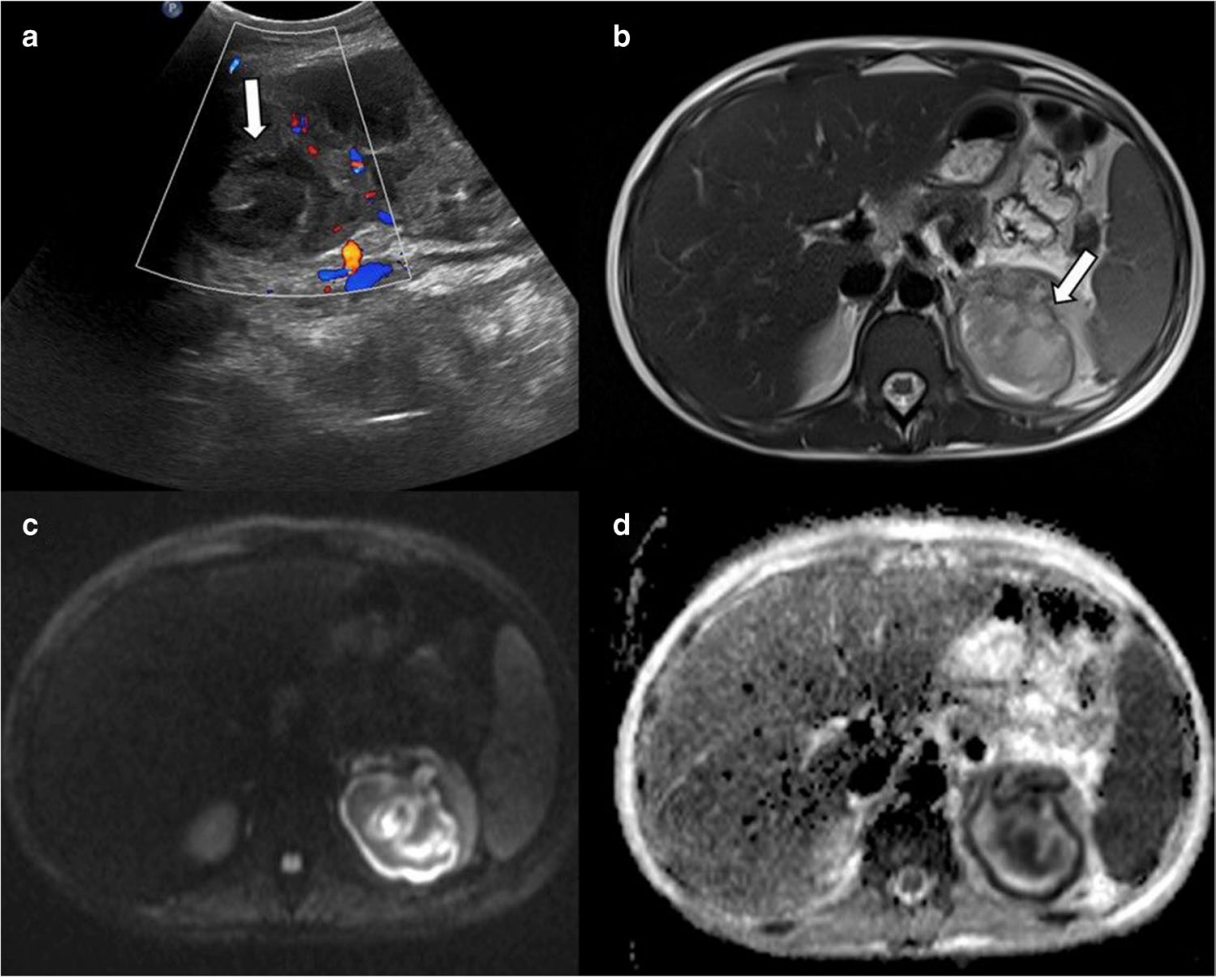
Renal abscess. A 7-year-old girl had fever and left upper quadrant pain. a Longitudinal ultrasound (US) image demonstrates a heterogeneous hypoechoic lesion in the upper pole of the left kidney, with no internal vascularity on color Doppler US (*arrow*). b Axial T2-weighted image reveals a mildly hyperintense lesion with a hypointense rim in the same region (*arrow*). c, d Diffusion-weighted imaging (*b* = 800 s/mm^2^) (c) and the apparent diffusion coefficient map (d) show restricted diffusion in the center and wall of the lesion, consistent with an abscess. The lesion was aspirated and *Stenotrophomonas maltophilia* was identified on the culture
